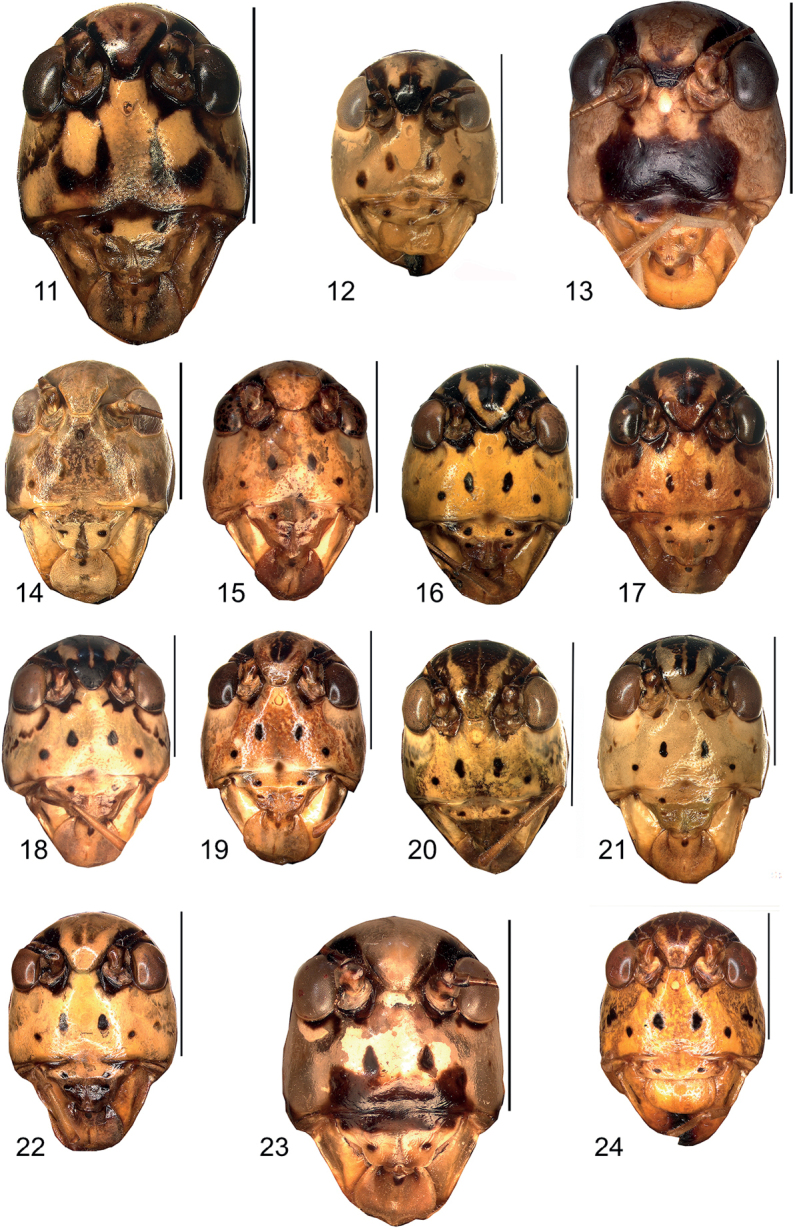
Colour pattern of head, frontal view 11*Eupholidopterasmyrnensis* ♀ Makrigiannis RMNH5014918 12*Eupholidopteragemellata* ♂ Mt. Idhi RMNH.INS1141843 13*Eupholidopterapallipes* ♂ paratype Mt. Lefka RMNH.INS1105313 14*Eupholidopteracretica* ♂ Mt. Lefka, Omalos RMNH.INS114838 15*Eupholidopteralatens* ♀ Mt. Lefka, Kalergi CT1987.047.04 16*Eupholidopteragiuliae* ♂ Argoules CT1995.011.18 17*Eupholidopterafrancisae* sp. nov. ♀ allotype Andikithira CT 2002.004.11 18*Eupholidopteraannamariae* ♀ Kato Zakros CT1995.020.03 19*Eupholidopteraastyla* ♀ Ano Viannos CT1987.024.03 20*Eupholidopteraferi* ♂ holotype Mt. Dikti, Katharo plain RMNH.INS1105297 21*Eupholidopteramariannae* ♀ Ag. Ioannis RMNH.5014906 22*Eupholidopteraforcipata* ♂ Mt. Idhi CT1987.044.03 23*Eupholidopteramarietheresae* sp. nov. ♂ paratype Mt. Dikti, FC1606 CT2000.096.01 24*Eupholidopterajacquelinae* ♂ holotype Gavdos CT2000.005.02. Scale bars: 5 mm.
